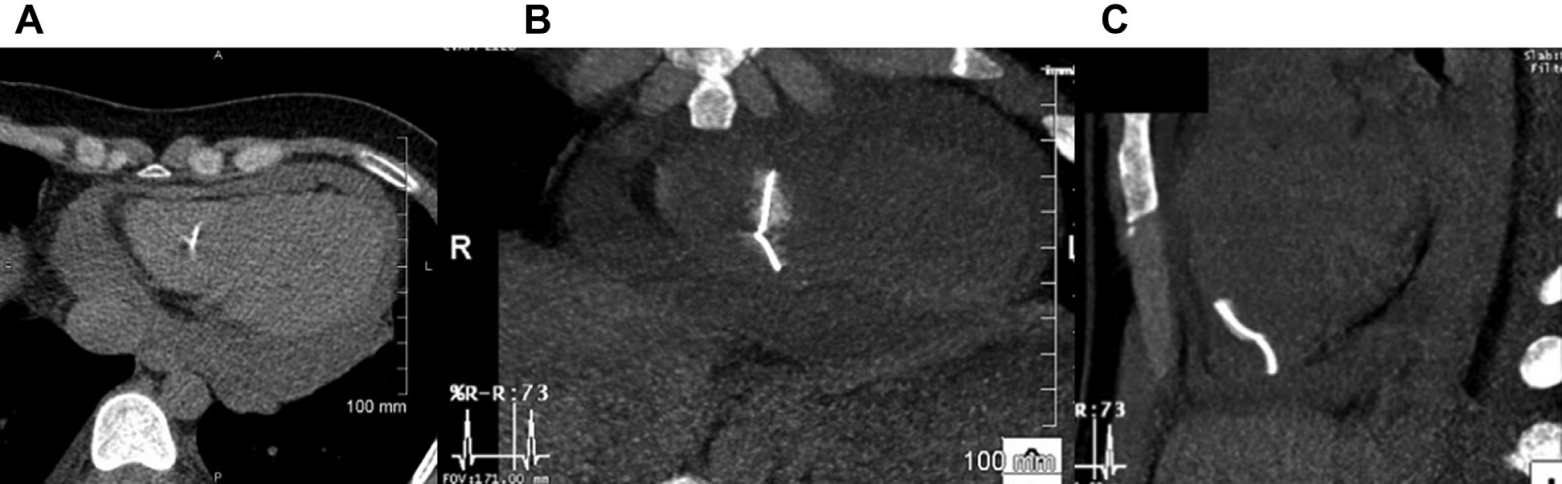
Cardiac Computed Tomography Noncontrast computed tomography (CT) images demonstrating (A) circumferential pericardial effusion and a linear metal density in the right ventricle. (B and C) Curvilinear reformatted images depicting the arrangement of 2 adjacent needles or 1 fractured needle with 1 end anchored to the right ventricular wall. R = right.
